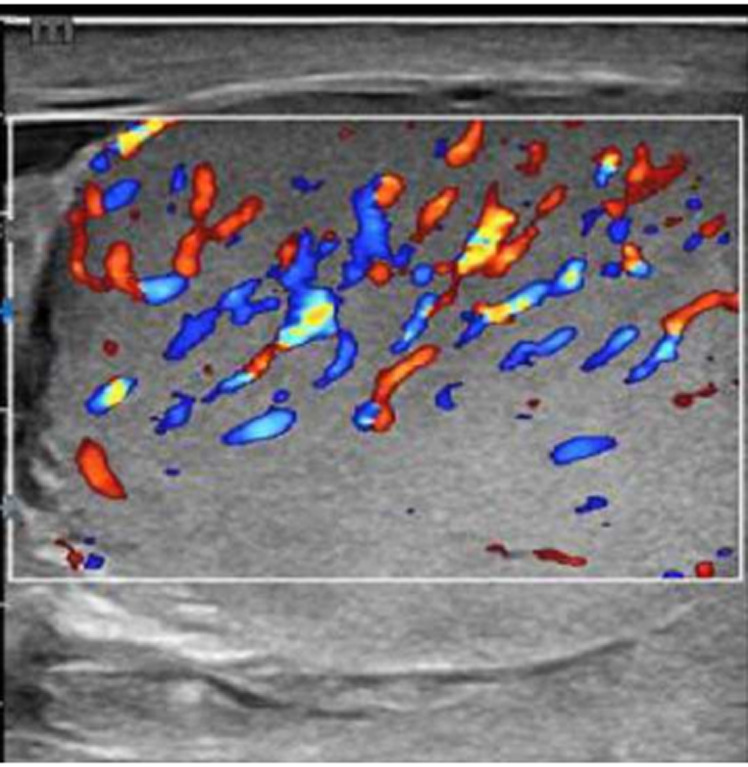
ultrasonography of the left testicle showing increased arterial vascularity in favour of epididymo-orchitis
